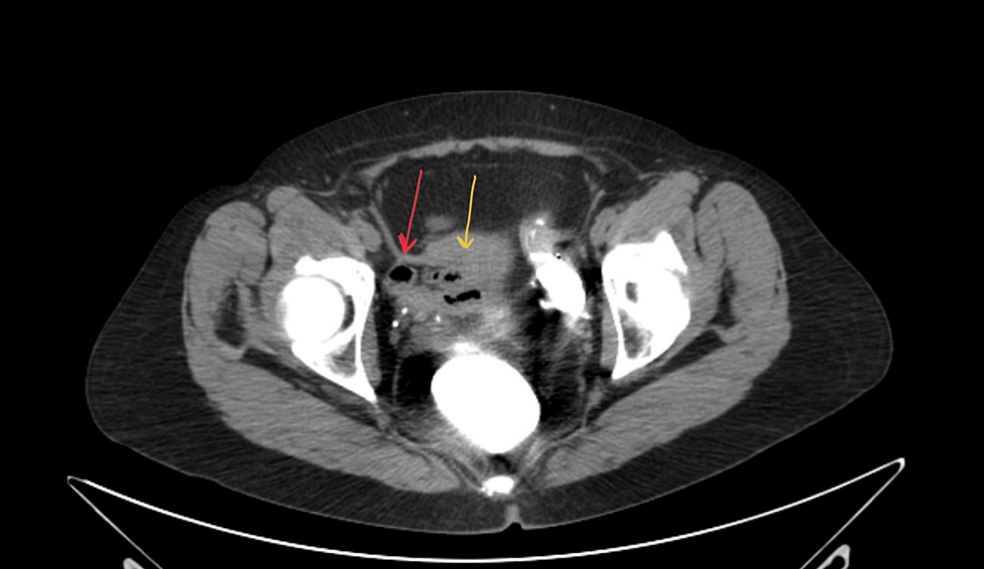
Axial CT scan with contrast of the pelvis shows the uterus (yellow arrow) and right fallopian tube (red arrow), which failed to show the fistula. CT: computed tomography
